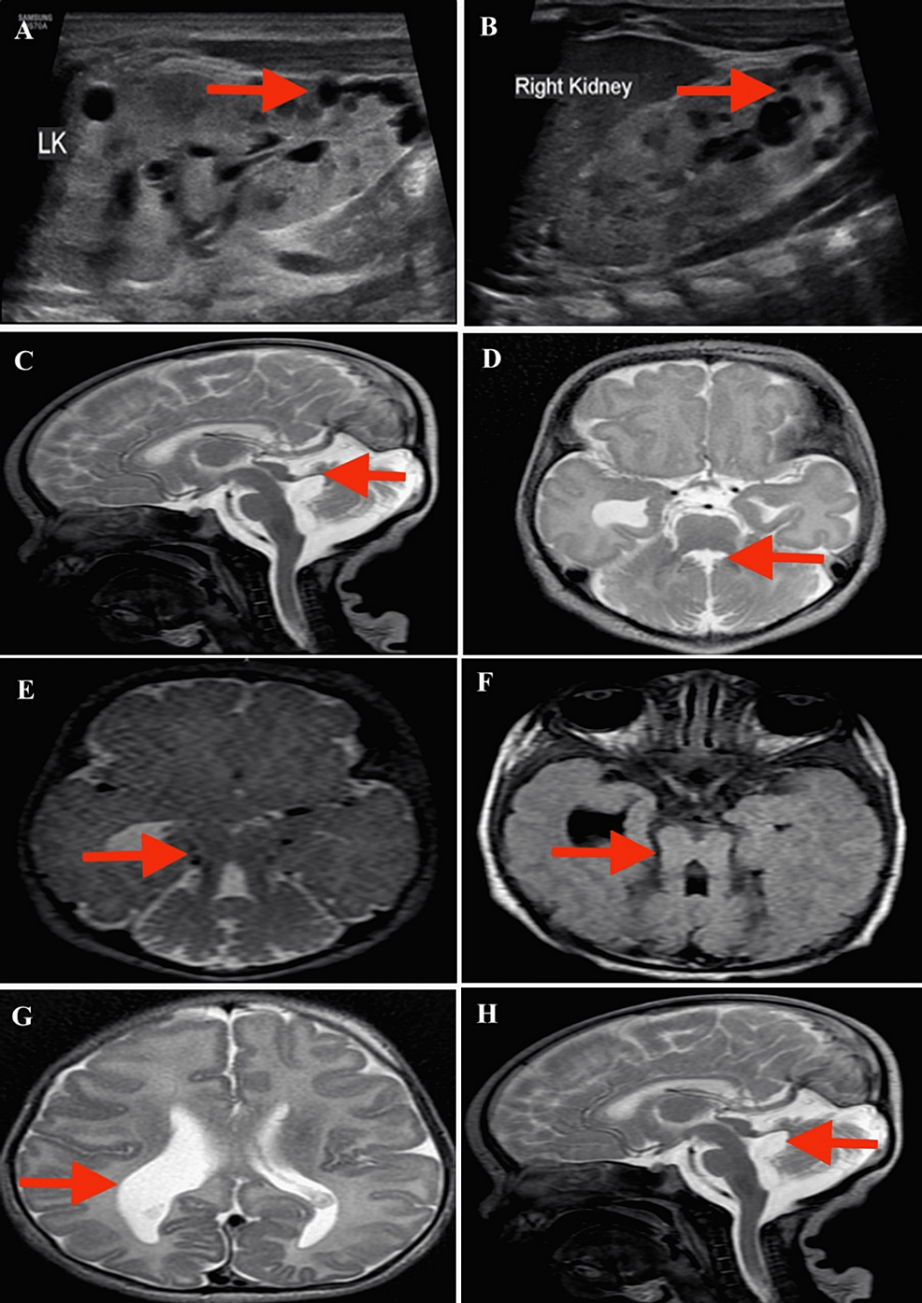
(A,B) USG of bilateral kidneys showed increased cortical echogenicity with reduced CMD and multiple small, simple cortical and medullary cysts. (C) Sagittal T2 MRI showed hypoplasia of the vermis and enlargement of the fourth ventricle with upward and posterior displacement of the fastigium. (D) Axial T2 MRI showed batwing appearance of the fourth ventricle. (E,F) Axial T2 and FLAIR MRI showed thick and elongated superior cerebellar peduncles with a deep interpeduncular fossa. (G) Axial T2 MRI showed asymmetrical dilatation of the right lateral ventricle. (H) Sagittal T2 MRI showed thinning of the corpus callosum. USG, ultrasonography; CMD, corticomedullary differentiation; MRI, Magnetic Resonance Imaging; FLAIR, fluid-attenuated inversion recovery
